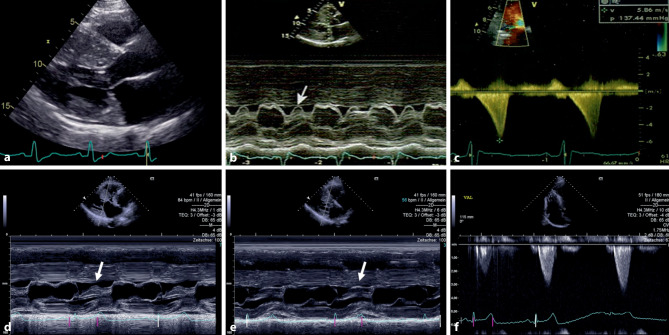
Echocardiographic features of left ventricular outflow tract obstruction. a–c patient with resting obstruction, d, e patient with provocable obstruction. a B-mode in parasternal long axis showing complete SAM at late systole. b M-mode in parasternal long axis showing complete SAM (*arrow*). c Dagger-shaped obstruction signal derived from continuous wave Doppler in apical 5‑chamber view. Bottom: patient with provocable obstruction. d M-mode in parasternal long axis showing incomplete SAM at rest (*arrow*). e M-mode in parasternal long axis showing incomplete SAM during Valsalva maneuver (*arrow*). f Obstruction signal derived from continuous wave Doppler in apical 5‑chamber view with increasing gradient during Valsalva maneuver. *SAM* systolic anterior motion
